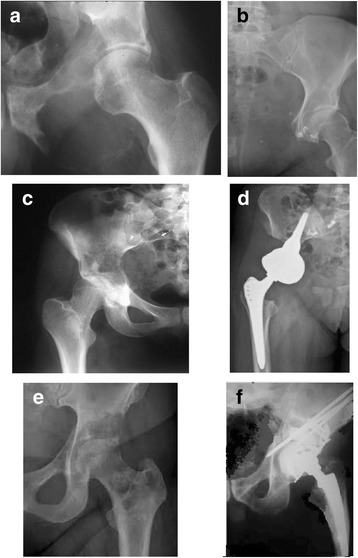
Preoperative and postoperative radiograph series of patients with metastatic pelvis tumours. (a) showed preoperative radiograph of metastatic renal cancer (type III involvement), and (b) after resection without bone reconstruction. (c) showed breast metastatic cancer in periacetabular area, and (d) after type II resection and Lumic prosthesis implantation. (e) showed periacetabular myeloma metastasis, and (f) Harrington bone reconstruction
